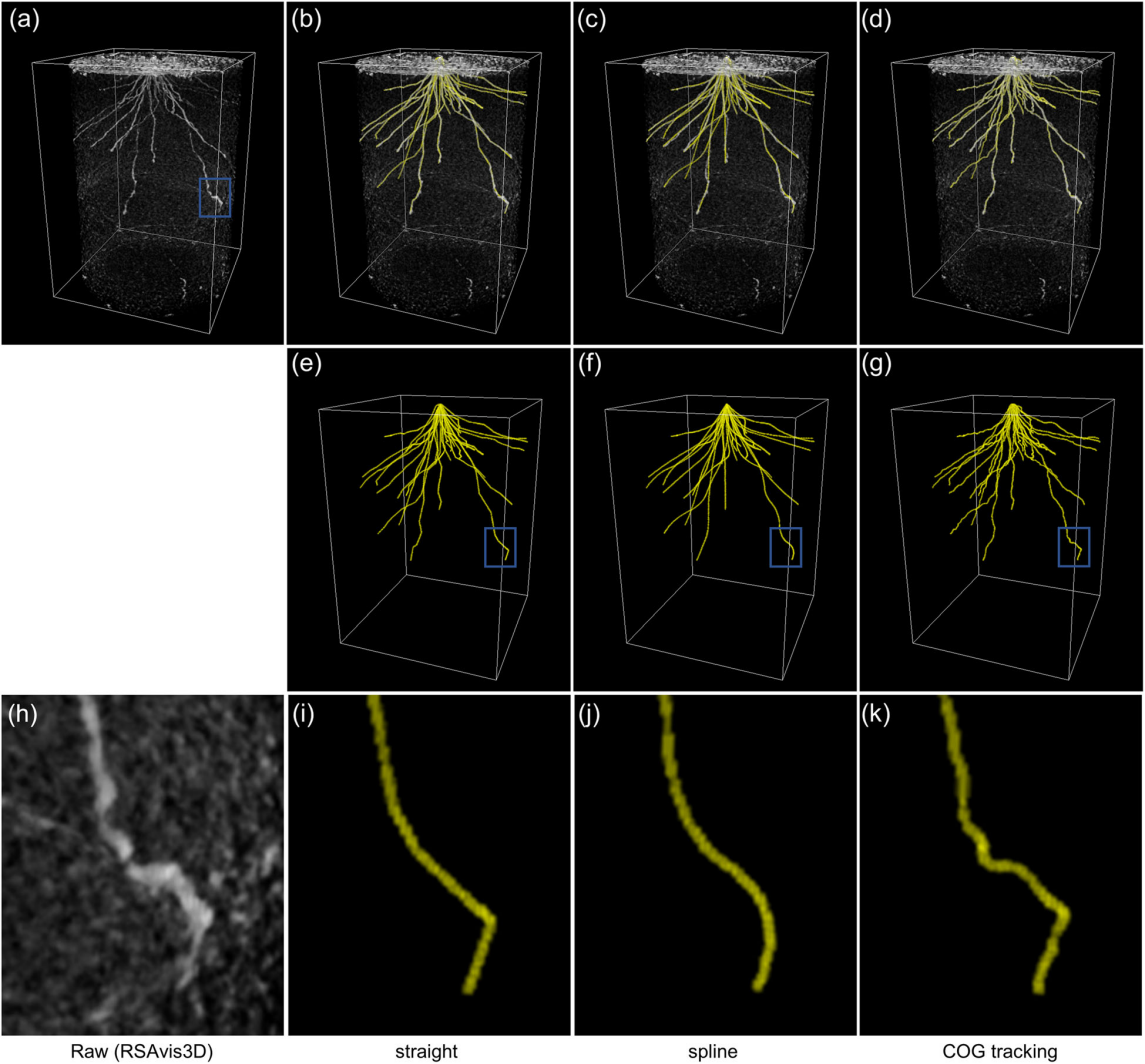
Comparison of tracing performance among the three vectorization methods. The CT images were processed by employing RSAvis3D and vectorized by applying RSAtrace3D. (a) 3D rendering of s roots of 26-DAS KP in an X-ray CT image. The X-ray CT image (a) was merged with the vectorized polylines by (b) “Straight,” (c) “Spline,” and (d) “COG tracking.” The vectorized polylines are colored in yellow. The vectorized polylines (e), (f), and (g) were isolated from those of (b), (c), and (d), respectively. The enlarged version of the interior, circled by the blue square, in (a), (e), (f), and (g) are shown in (h), (i), (j), and (k), respectively. The rectangle indicates (width) 180 mm × (depth) 180 mm × (height) 258 mm
